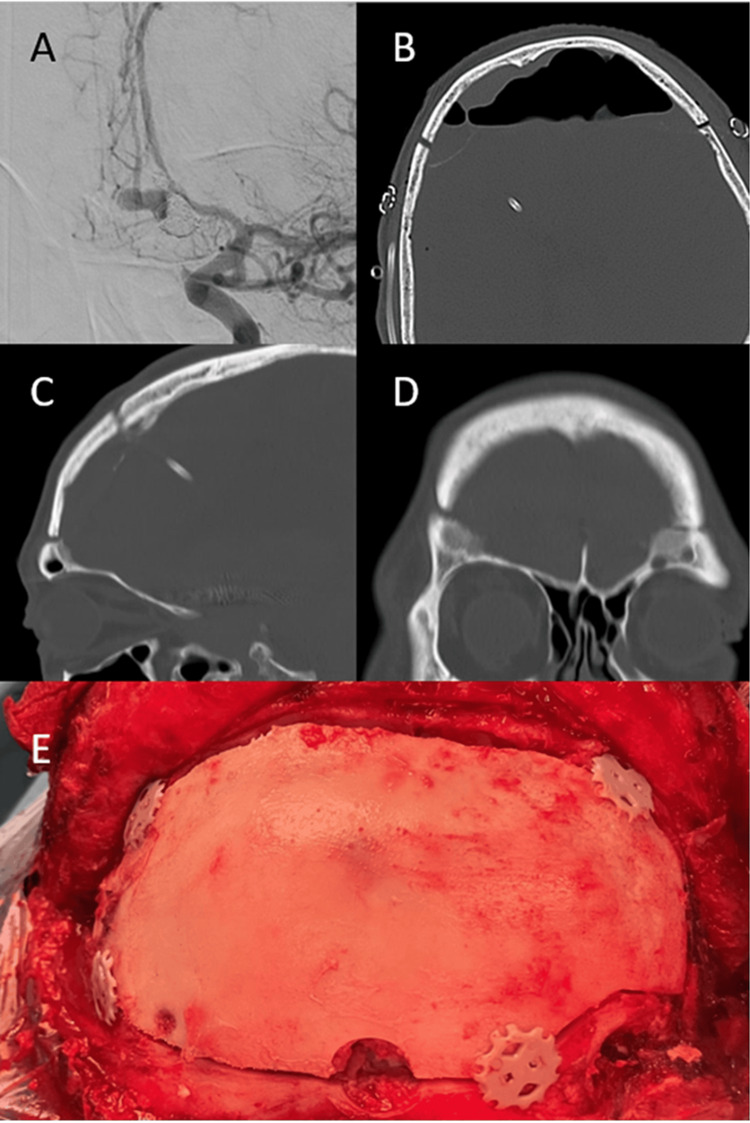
A 43-year-old man undergoing clipping of an anterior communicating artery aneurism (A) Pre-operative arteriography image: anteroposterior view showing the rechannelled anterior communicating artery aneurism. (B) Twenty-four-hour post-operative CT scan image: axial view of the bone. (C) Seven-month post-operative CT scan image: sagittal reconstruction view of the bone. (D) Seven-month post-operative CT scan image: coronal reconstruction view of the bone. (E) Surgical view after microsurgical clipping and fixation of the bone flap with Cranial LOOP™.
